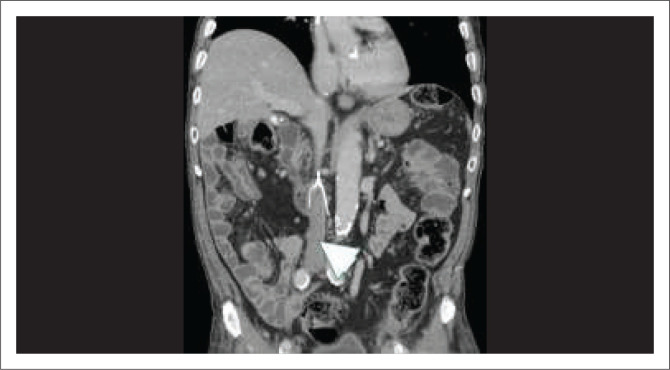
The coronal contrast-enhanced CT image of the abdomen showed an inferior vena cava (IVC) filter in place with a non-enhancing intraluminal filling defect (arrowhead) in the IVC extending all the way to the level of the IVC filter.
